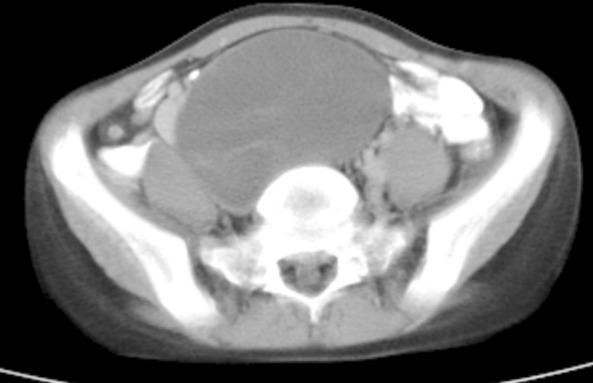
Computed tomography scan shows a mass arising in the retro peritoneum.
